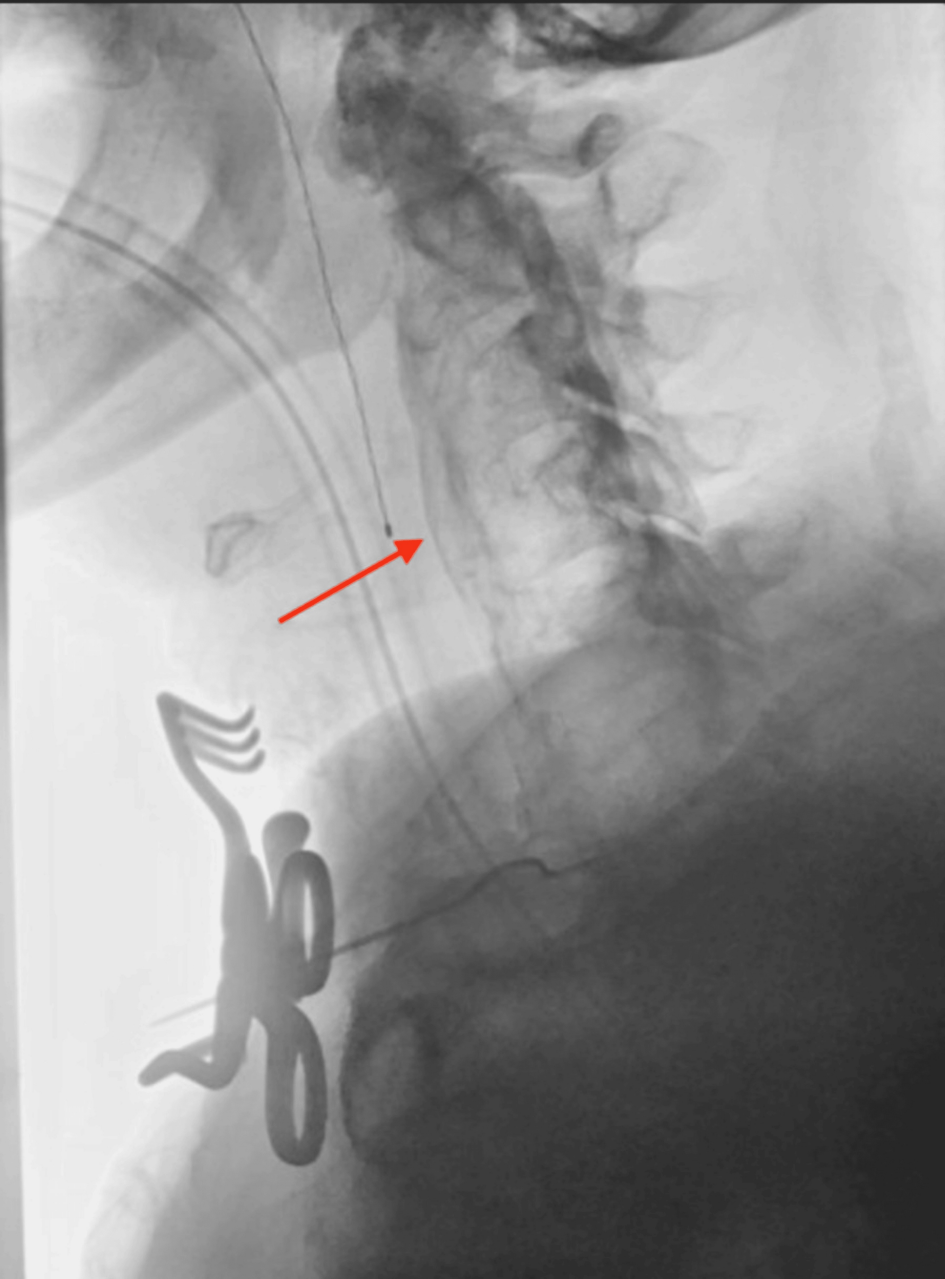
X-ray demonstrating causing posterior displacement of the trachea and esophagus (arrow).
